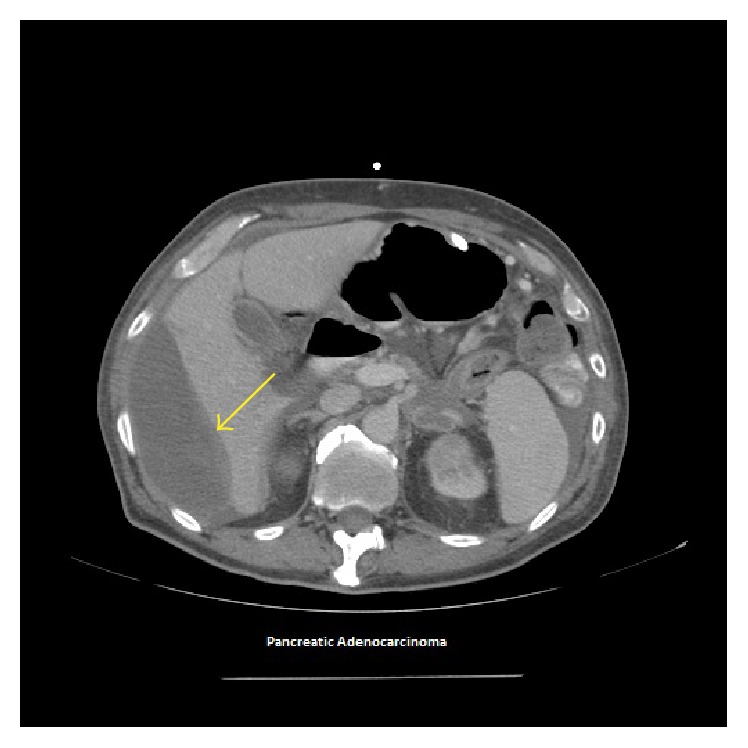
Axial contrast enhanced CT obtained 4 months after study in Figures 1 and 3 showing decreasing size of subcapsular liver collection.
